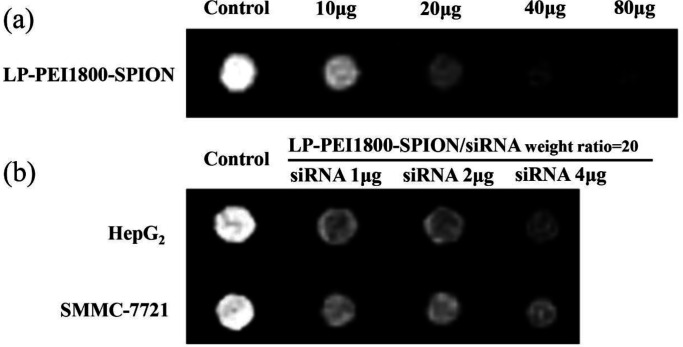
Images T2-weighted MRI with (a) LP-PEI1800-SPION at various weight ranging from 10 to 80 μg in PBS and (b) LP-PEI1800-SPION/siRNA-Luc at various weight ratios from 1 to 4 μg of siRNA-Luc in HepG2 and SMMC-7721 cells (TE = 117 ms. The cells with no treatment was used as a control)
